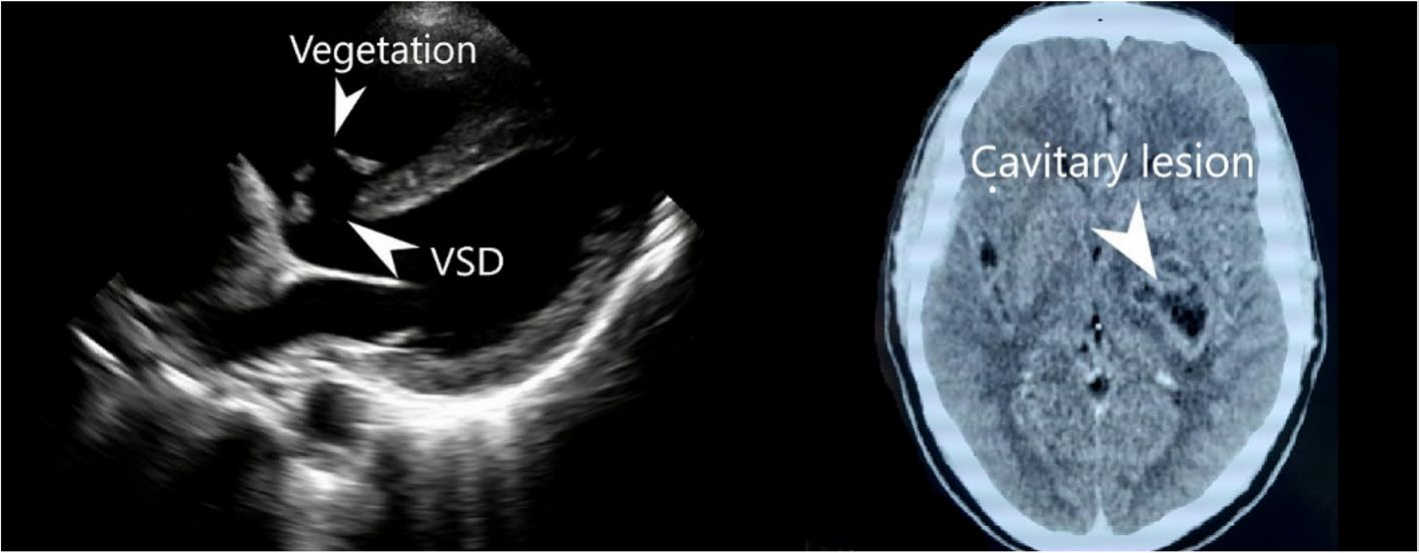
Cardiac POCUS revealing valvular vegetation (left) and brain CT with findings consistent with brain abscess (right)
